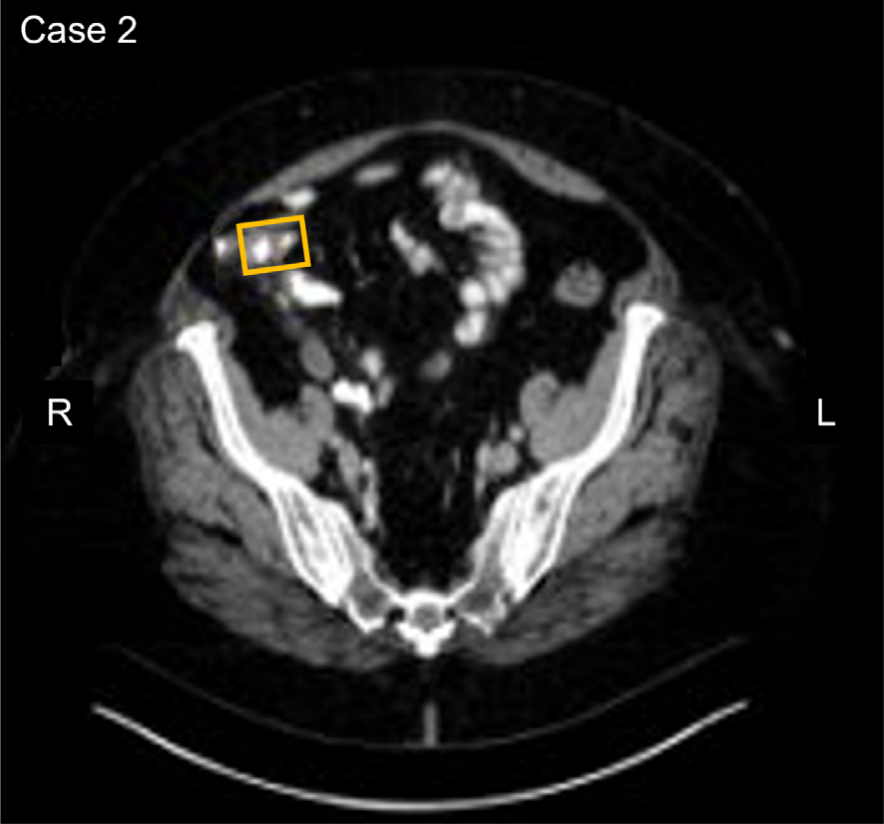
Participant two CT image. The area in the yellow box shows an appendix measuring 1.3 cm and displaying internal fluid density without surrounding inflammatory changes.
